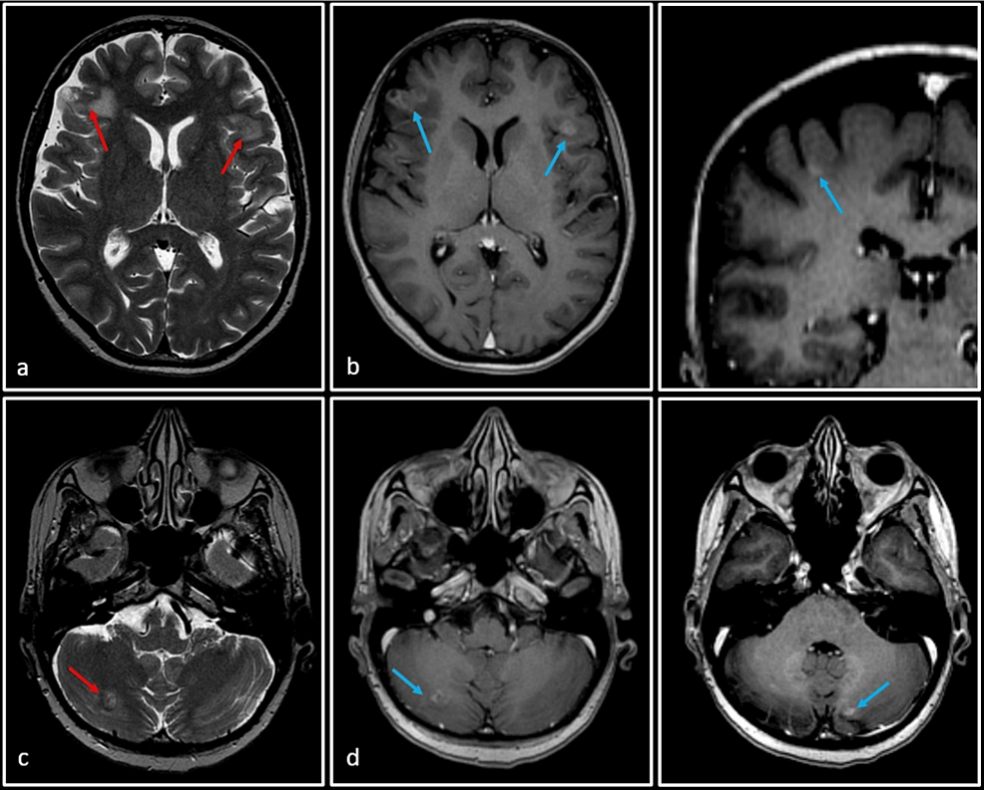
Follow-up MRI of the patient T2-weighted images (a and c) and post-Gadolinium T1-weighted images (b, d, e and f) show two ill-defined lesions in bilateral frontal lobes and one in the right cerebellar hemisphere (red arrows), with associated perilesional edema. The lesions are better appreciated on post-Gadolinium T1-weighted images (blue arrows), where a total of five lesions are seen: two in bilateral frontal lobes, two in bilateral cerebellar hemispheres, and the smallest one just inferior to the central sulcus on the right side at the grey-white matter junction. All these lesions were consistent with known metastases (post-whole brain radiation therapy).
